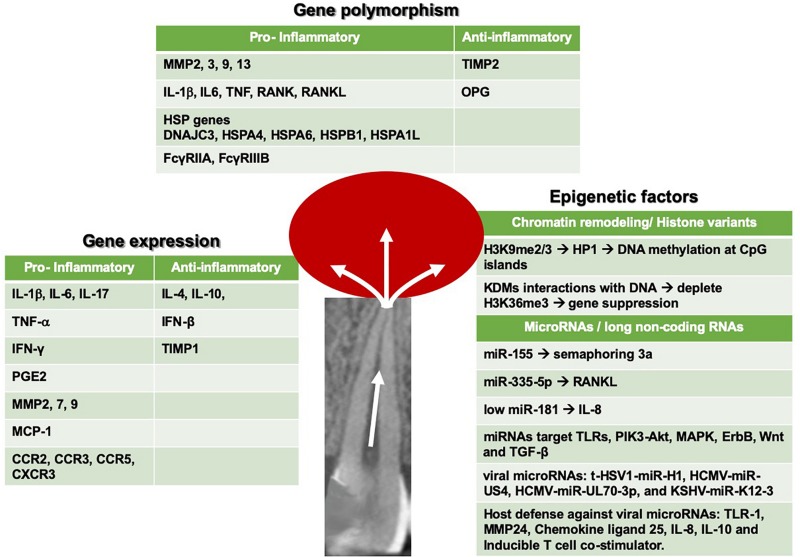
Summary of seminal gene expression, gene polymorphism and epigenetic mediators, and pathways that have been recently reported in pulp and periapical inflammation.
